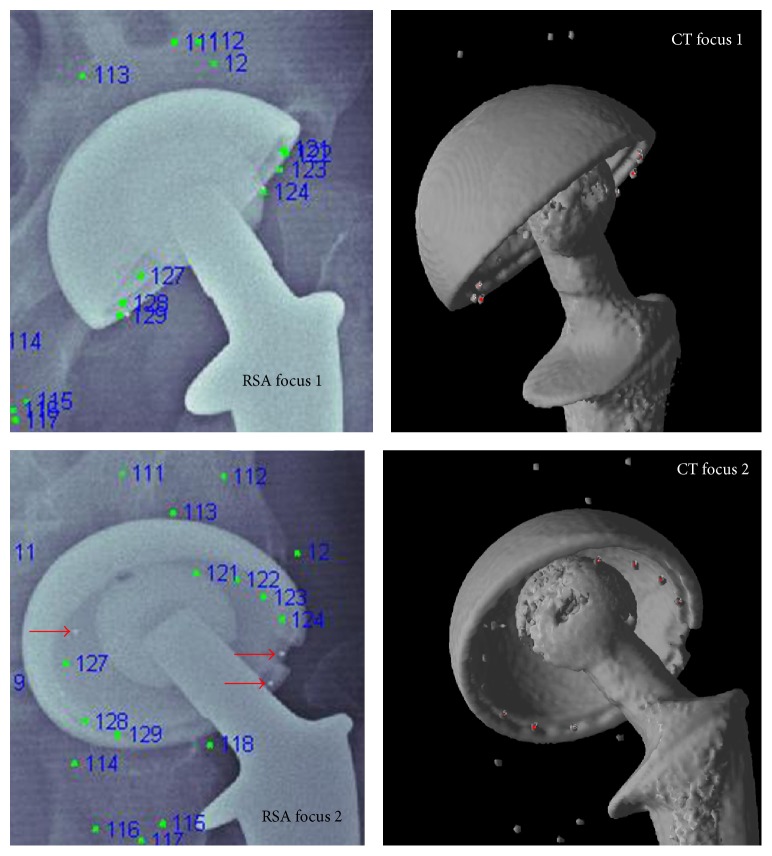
The isosurface level for the CT volumes is set so that only metal is visible. The image of the 3D CT volume is rotated until it matches focuses 1 and 2 of the RSA radiographs. The tantalum beads in the periacetabular bone are numbered in the CT volume in the same sequence as in the RSA radiographs. Via rigid body transformation these bone marker coordinates from the RSA volume and CT volume are matched. Thereafter the tantalum beads of the liner in the CT volume are marked in the same way. Markers visible in only one of the RSA foci (red arrows) are excluded even in the CT volume.
